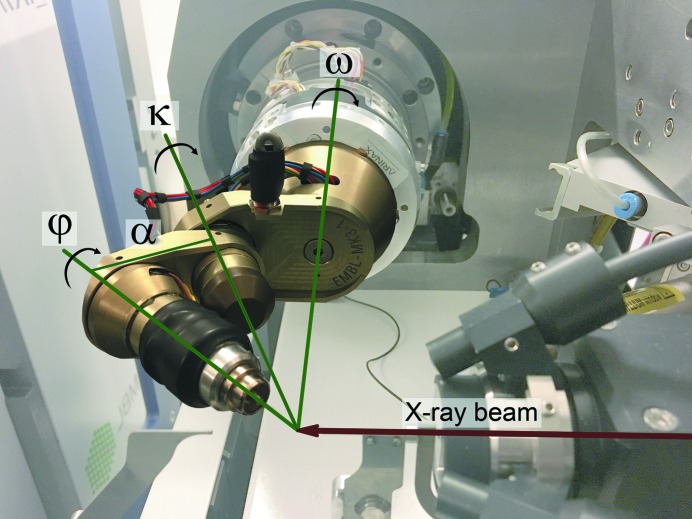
Experiment setup on the ID30B beamline at the ESRF (McCarthy *et al.*, 2018 ▸) with a miniKappa goniometer. The rotation axes are mounted in the following order, Omega (Ω), Kappa (K) and Phi (Φ). The angle alpha (α) between Kappa and Phi is nominally 24° by design. In the captured goniometer settings, Kappa is open by 180°, so Phi and Omega are separated by 2α. A sample is mounted in a loop at the end of a magnetically held pin on the Phi axis for positioning in the X-ray beam.
